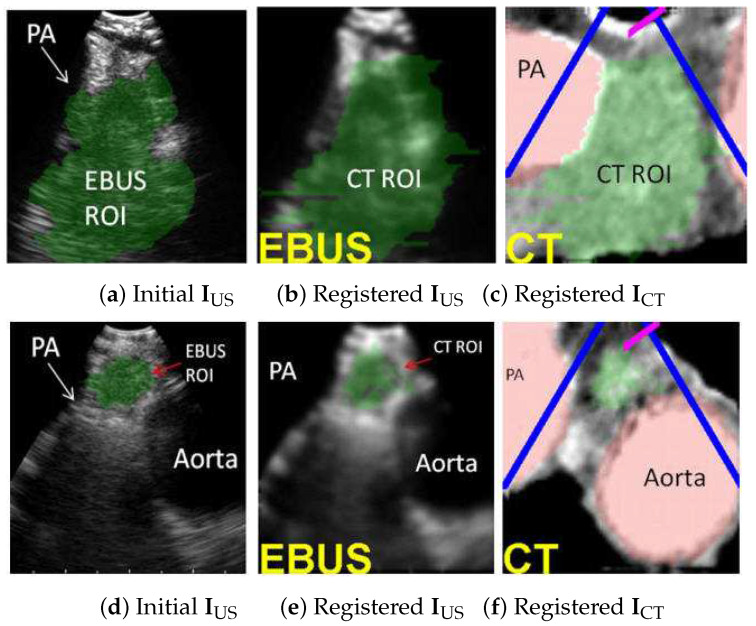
CT-EBUS registration examples. (a–c) Station 4L node for case 20349-3-84. (d–f) Station 4L node for case 21405-108. Parts (a,d) show the automatically segmented ROI in EBUS frame $I_{\mathrm{US}}$ using [42]. Parts (b,e) show the registered CT-based predefined ROI superimposed on $I_{\mathrm{US}}$. Parts (c,f) depict the CT-based virtual EBUS view $I_{\mathrm{CT}}^{p_{o}}$ after registration. In all views, the green region corresponds to the lymph node, whereas the red regions represent major vessels (PA = pulmonary artery).
